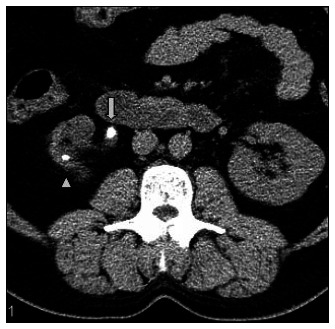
Ureteral fat stranding. Axial slice in noncontrast helical computed tomography (NCT). Stone identified in the proximal right ureter (arrow), associated with ureteral wall edema (tissue rim sign) and perinephric fat stranding. A stone in the kidney is also identified (arrow head).
